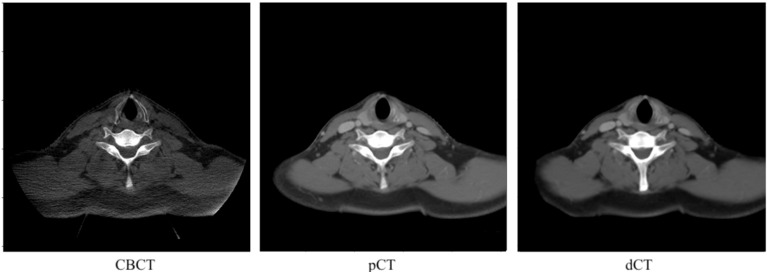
Examples of CBCT, pCT, and dCT images of the same slice.
